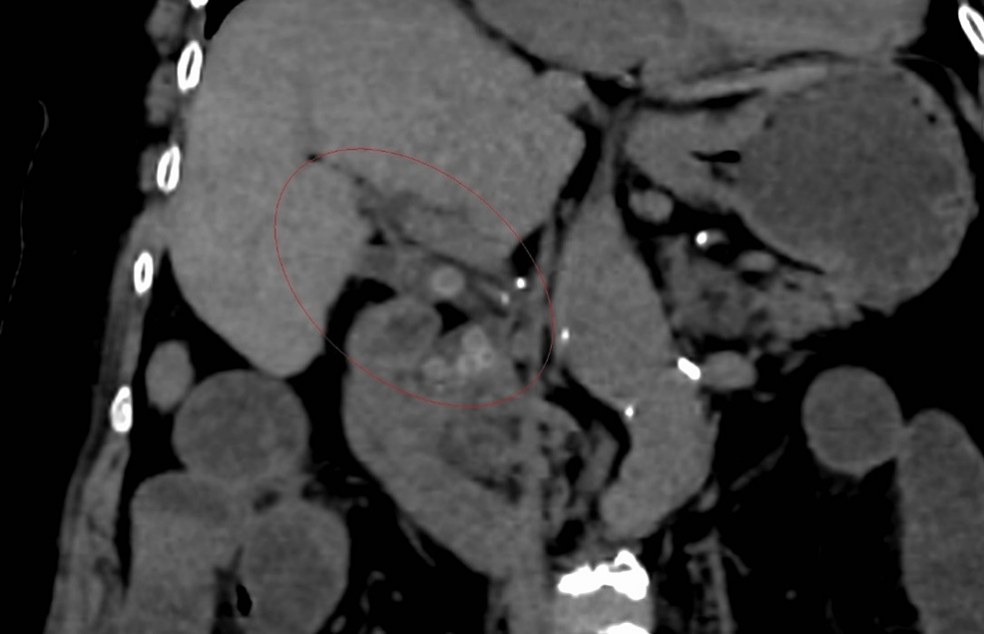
Non-contrast-enhanced computed tomography showing a cholecystoenteric fistula and multiple gallstones (red circle)
